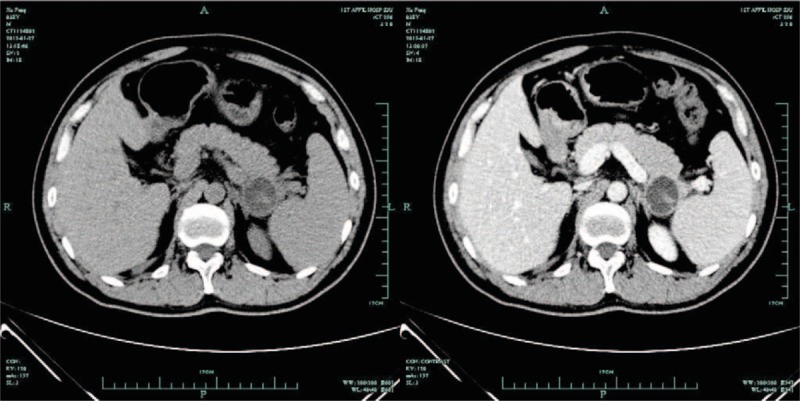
CT scans showed a cystic lesion in tail of pancreas.
